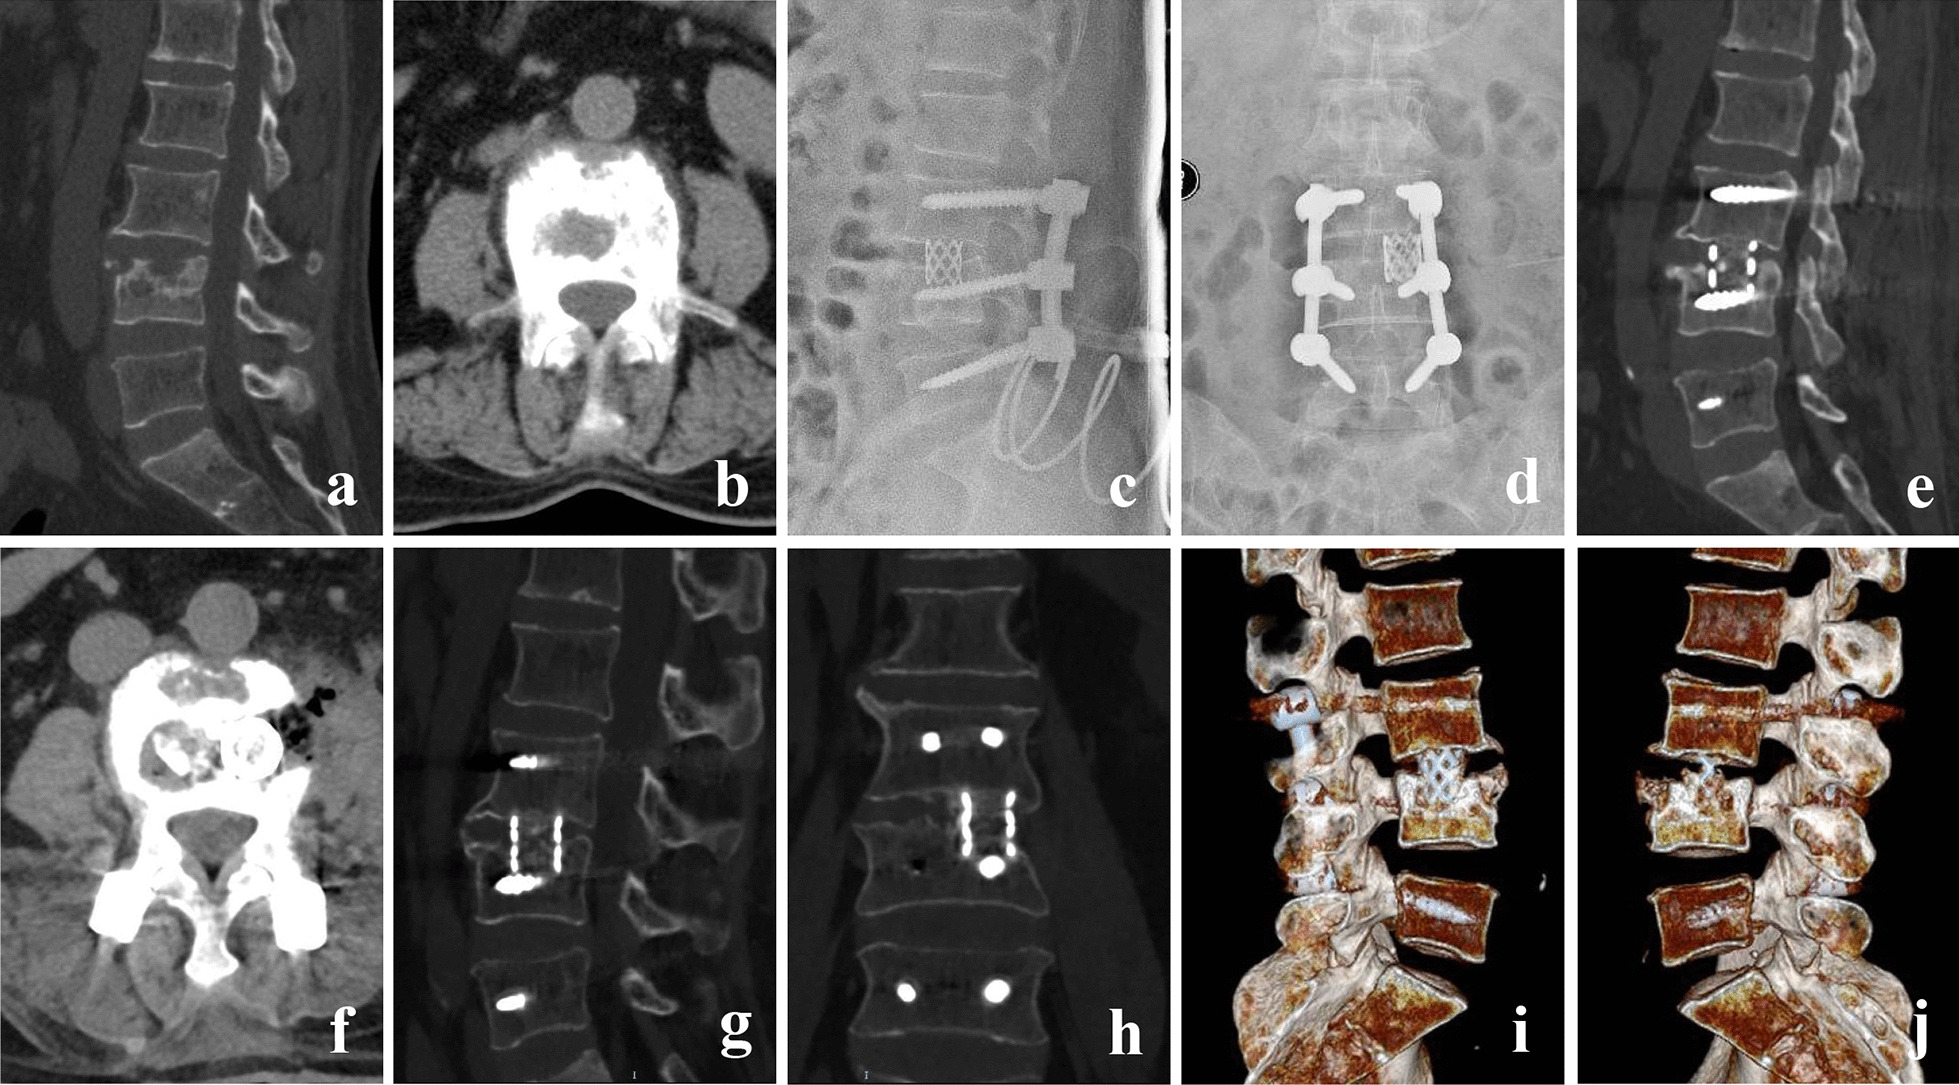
A 64-year-old female with L3-4 TB. (a, b) Preoperative CT showed that L3 and L4 vertebra body and the intervertebral disc were destroyed. (c, d) Postoperative X-ray. (e, f) CT at 3 months postoperative showed good location of titanium cage and posterior instrument. (g, h) CT at 6 months postoperative showed bone fusion between L3 and L4. (i, j) 3D-CT at 18 months postoperative showed good location of titanium cage and posterior instrument
